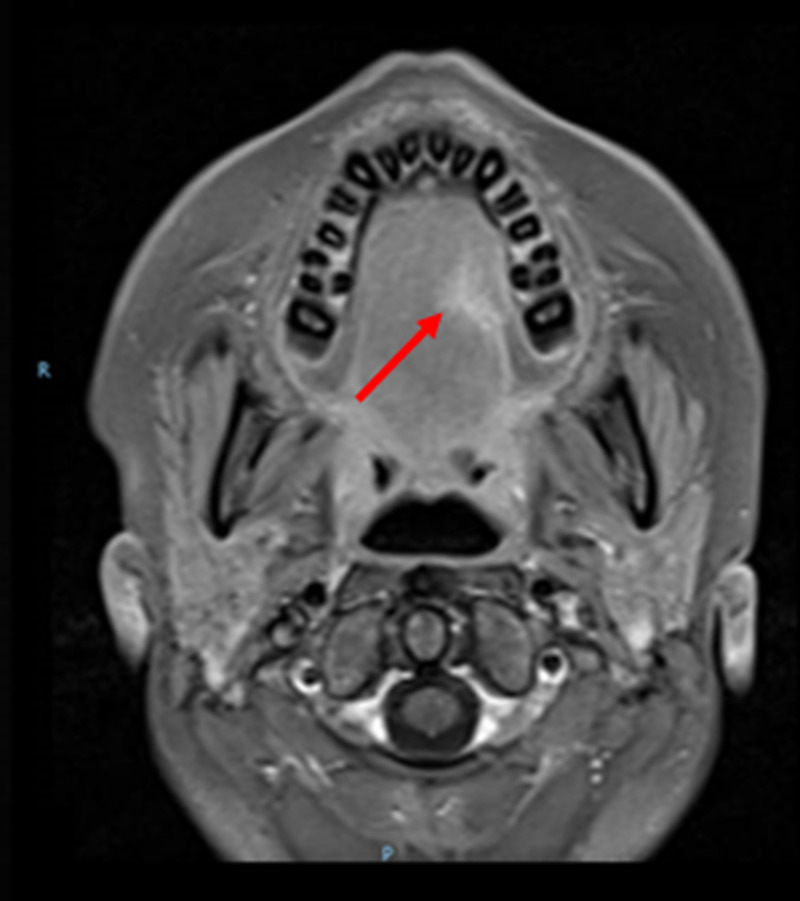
Post-treatment MRI. Post-treatment MRI shows the scar where the tumor was, with no signs of tumor relapse.
